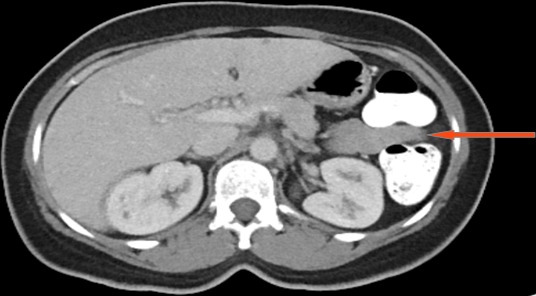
Post-surgery axial abdomen CT: shows several splenules, no spleen (red arrow)
